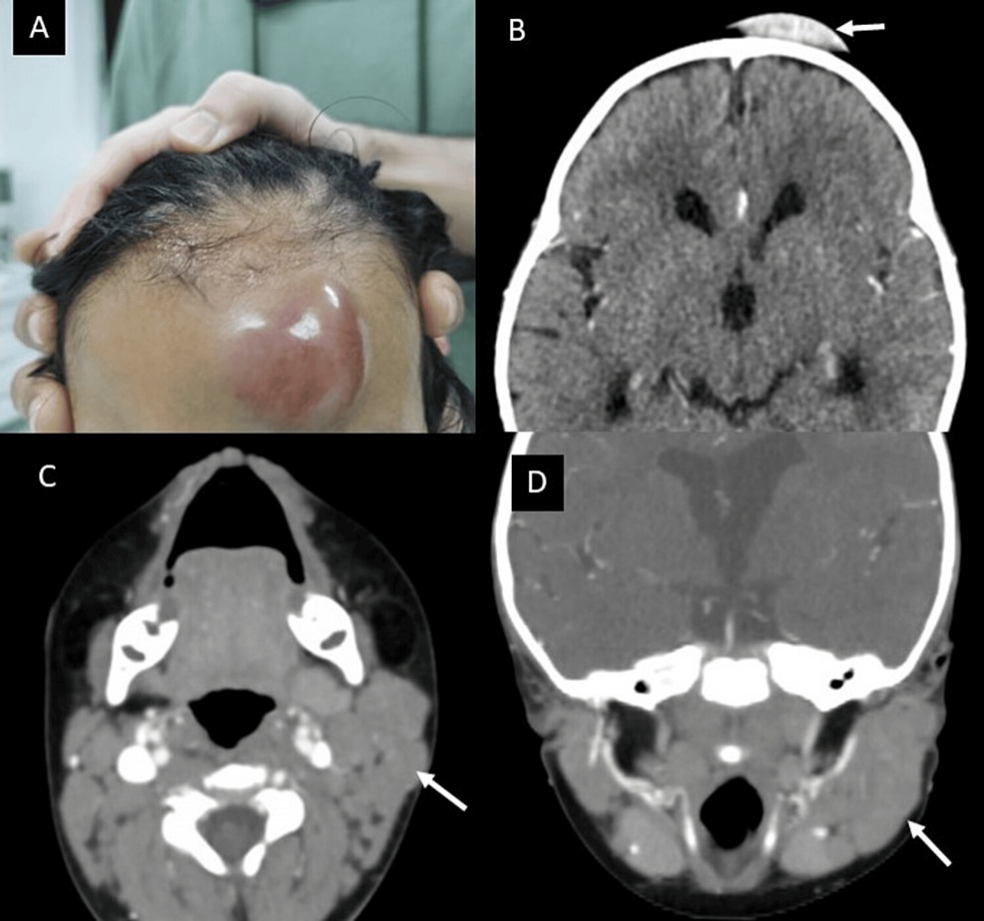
(A) Photograph of a violet frontal mass on the left side. (B) Contrast-enhanced axial head CT scan showing a mass in the left frontal epicranial soft tissues with homogeneous enhancement after contrast administration (white arrow). (C) Contrast-enhanced neck CT scan in axial section showing lymphadenopathies in stations IIa and IIb on the left side (white arrow). (D) Contrast-enhanced neck CT scan in coronal section showing left parotidomegaly (white arrow).
